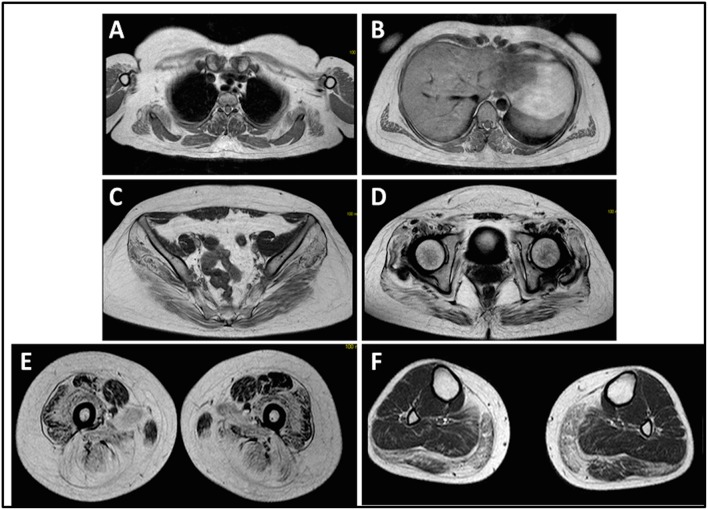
Proband muscle MRI: involvement of upper limb girdle and paravertebral muscles (A,B), involvement of lower limb girdle and posterior compartment of the thigh (C–E, mostly the glutei) with relative sparing of the sartorius and gracilis, and involvement of the posterior compartment of the leg (F, mostly gastrocnemius medialis/soleus).
